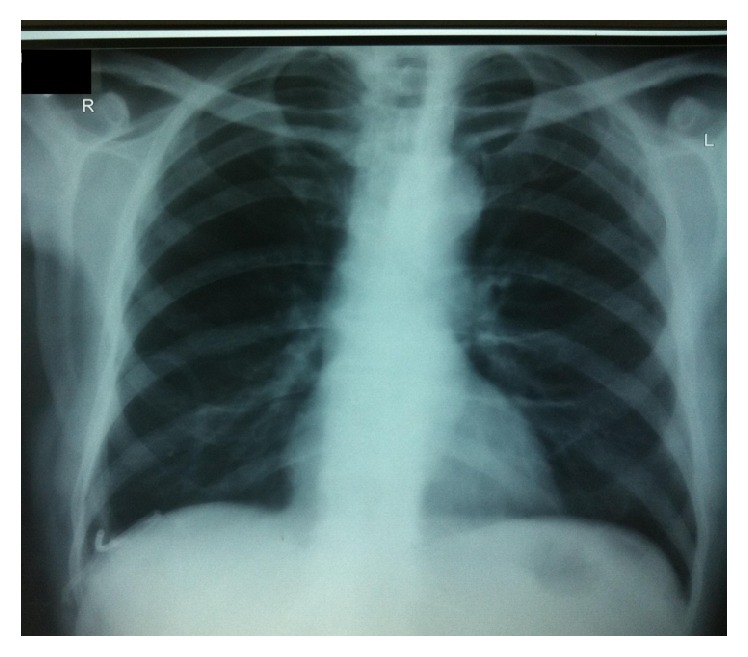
Chest X-ray of the same patient showing pigtail catheter in place with no effusion.
